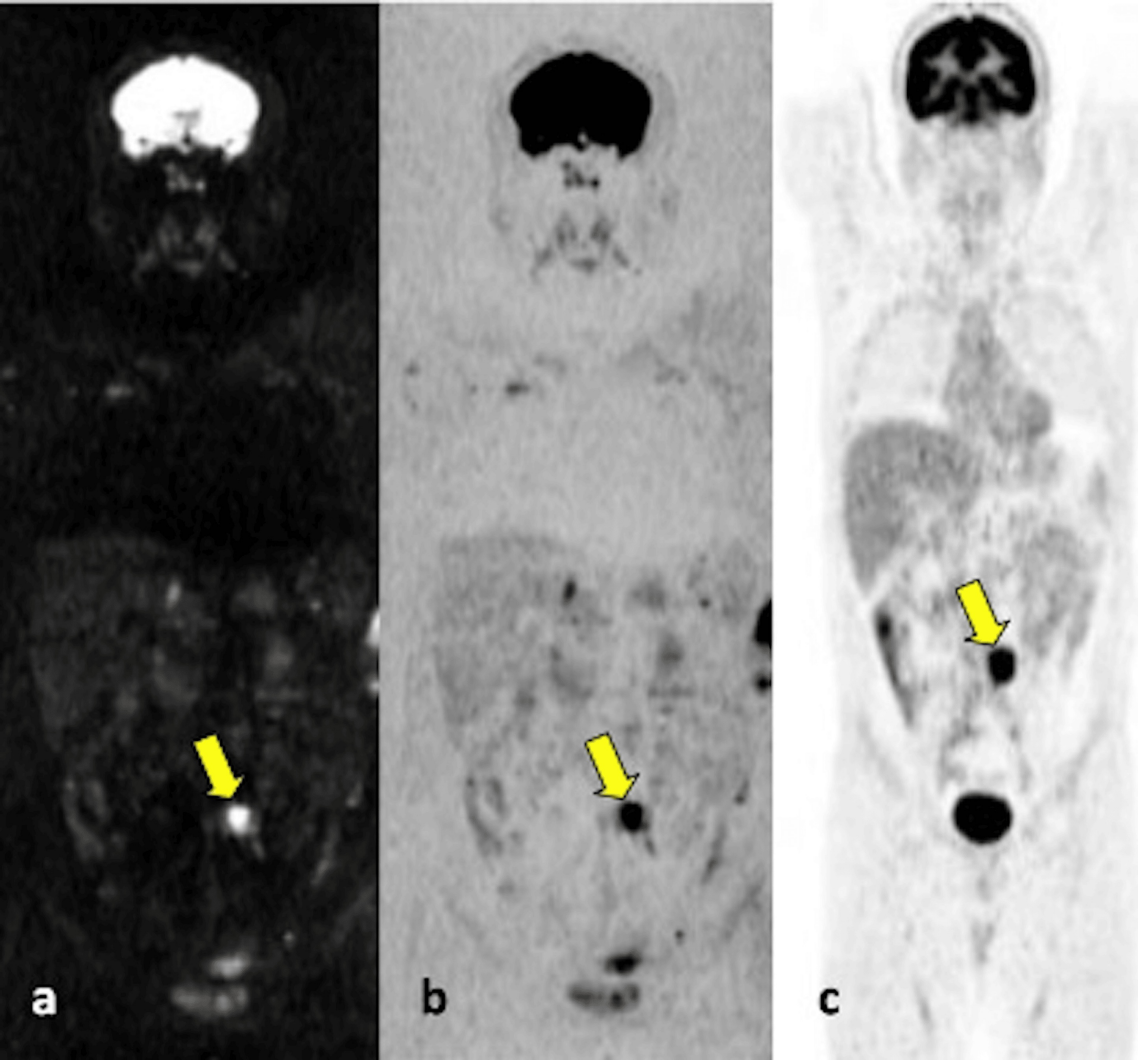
Coronal MPR (a) and inverted (b) whole-body DWI examinations of a 44-year-old male patient diagnosed with seminoma show an increased signal in the left para-aortic enlarged lymph node in the abdomen. Coronal PET (c) images reveal intense 18F-FDG uptake in the enlarged lymph node in the same region. MPR: multiplanar reconstruction, DWI: diffusion-weighted imaging, PET: positron emission tomography, 18F-FDG: fluorine-18 fluorodeoxyglucose.
